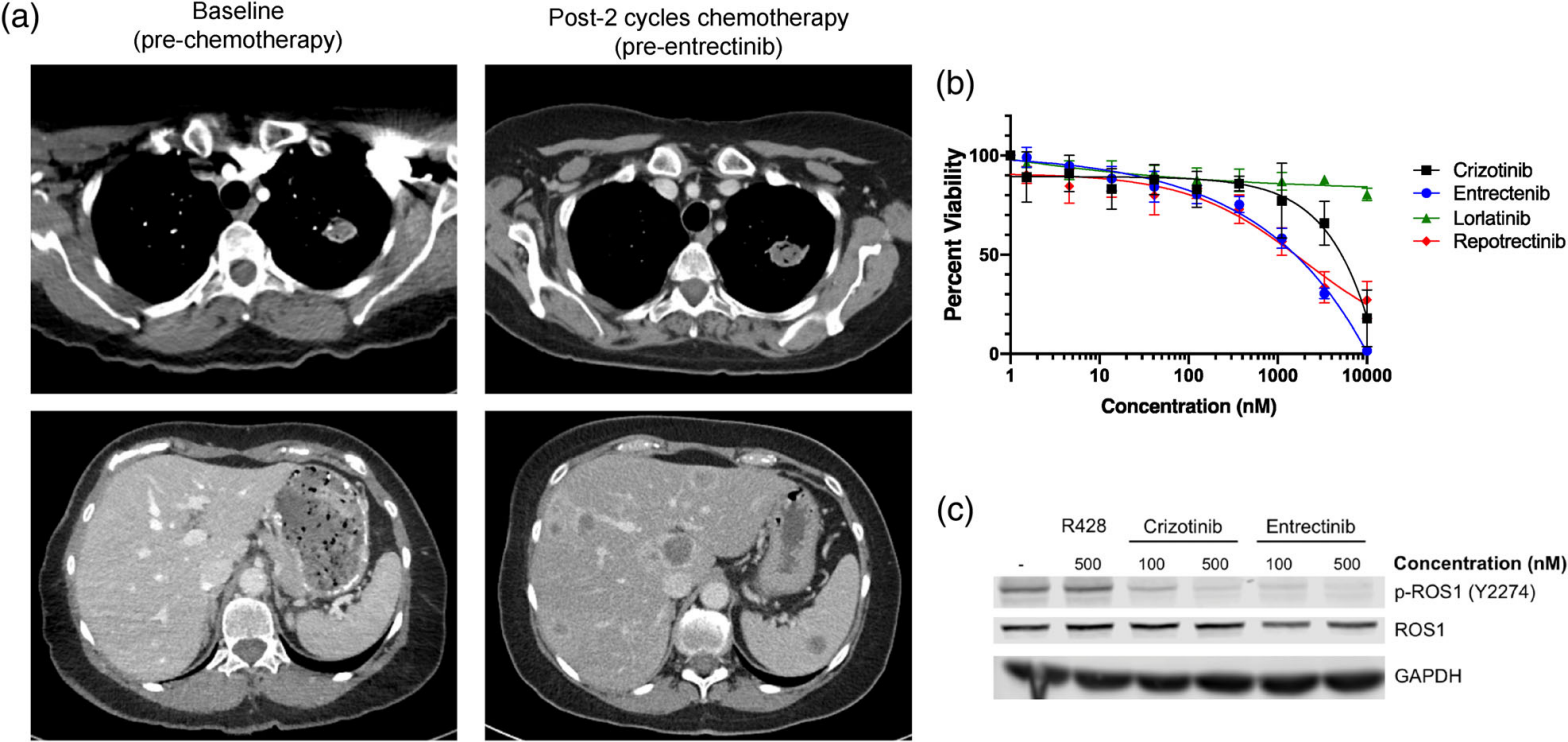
Derivation of a ROS1 inhibitor resistant cell line from a patient with intrinsic resistance. (a) Computed tomography (CT) scan of the patient prior to and after two cycles of chemotherapy. (b) Cell viability analysis with ROS1 inhibitors. CUTO33 cells were treated with the indicated concentration of drugs for 72 h and proliferation was measured by MTS assay. Showing the mean+/− SD, *n* = 3 biological replicates. (c) Inhibition of ROS1 phosphorylation. CUTO33 cells were treated with DMSO or the indicated concentrations of ROS1 inhibitors for 2 h prior to cell lysis and protein analysis by western blot. Representative images, *n* = 2.
